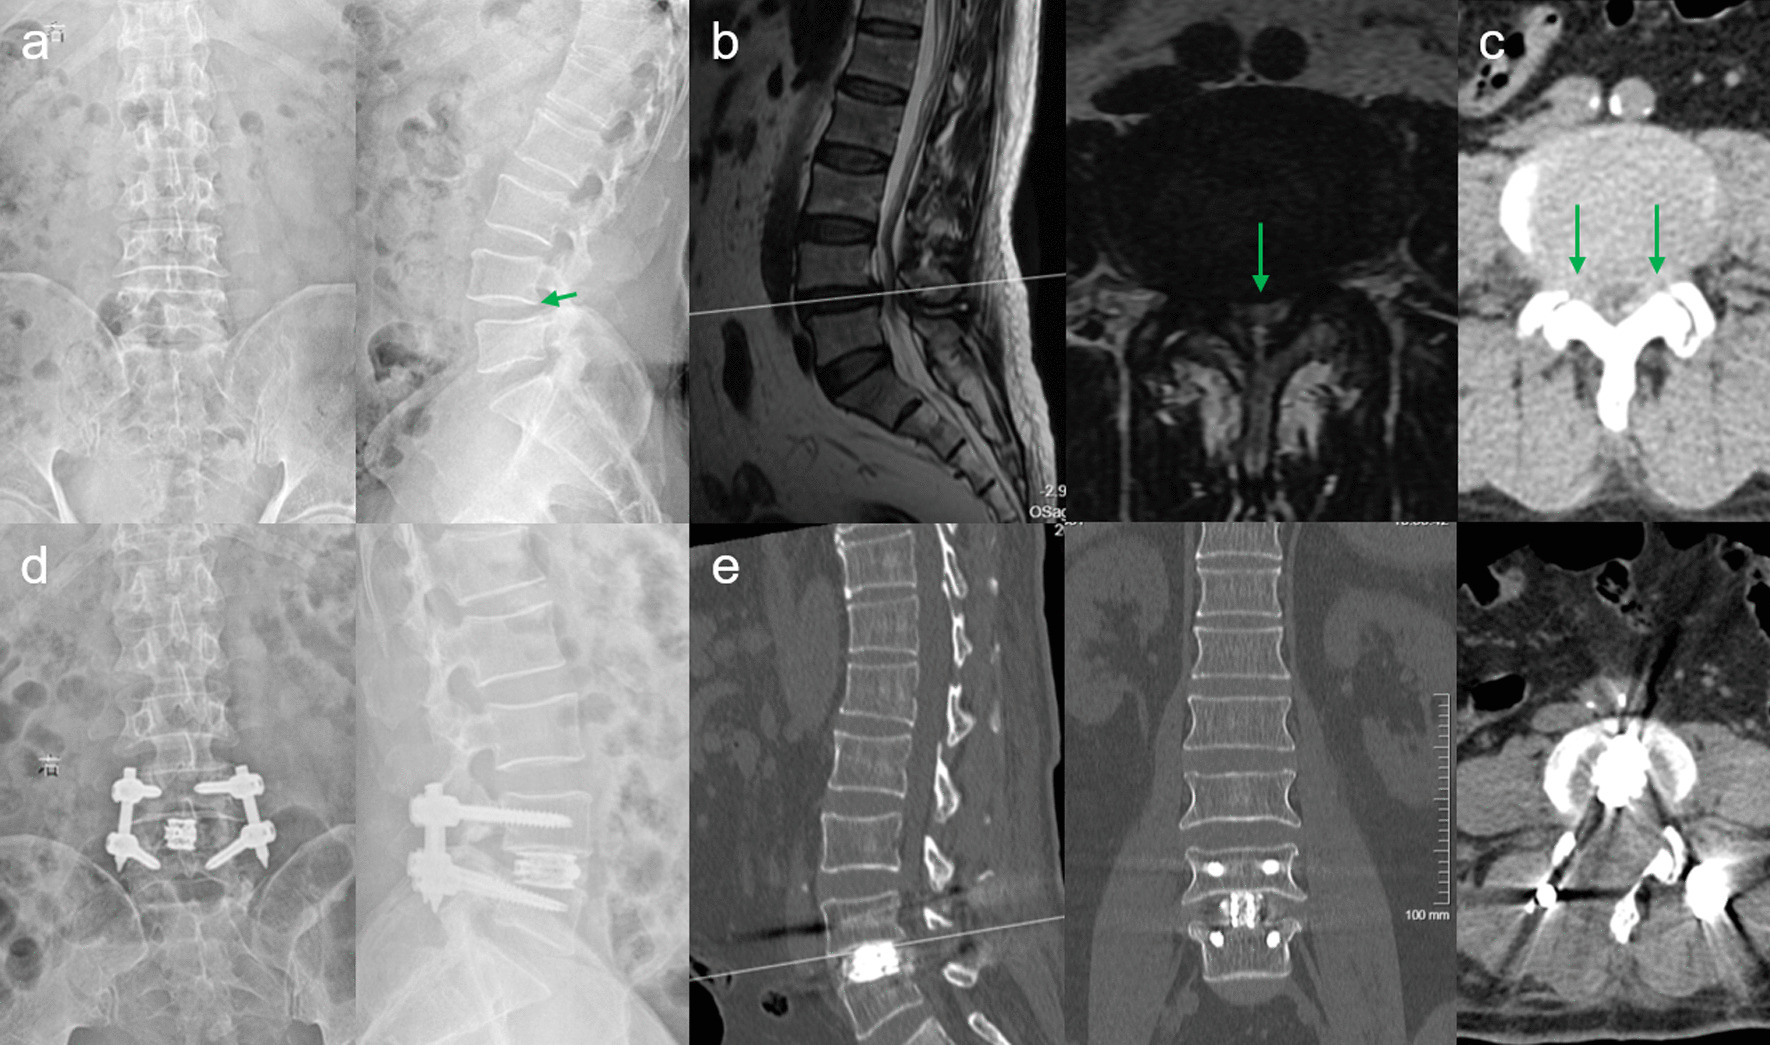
A 68-year-old male patient with severe intermittent claudication in both legs underwent PE-PLIF combined with the ‘Non-touch Over-Top’ technique. a preoperative AP X-ray fluoroscopy, and the green arrow showed I° spondylolisthesis at L_4-5_ segment. b preoperative MRI, the green arrow showed central canal stenosis at the L_4-5_ segment. c preoperative axial CT, and the green arrows showed bilateral LR stenosis at the L_4-5_ segment. d 1d postoperative AP X-ray fluoroscopy showed solid fixation of the implant and the successful reduction of the L_4-5_ segment. e 3d postoperative CT clearly demonstrated adequate spinal canal decompression. *PE-PLIF* percutaneous endoscopic posterior lumbar interbody fusion, *AP* anterior–posterior, *ULBD* unilateral laminotomy for bilateral decompression
